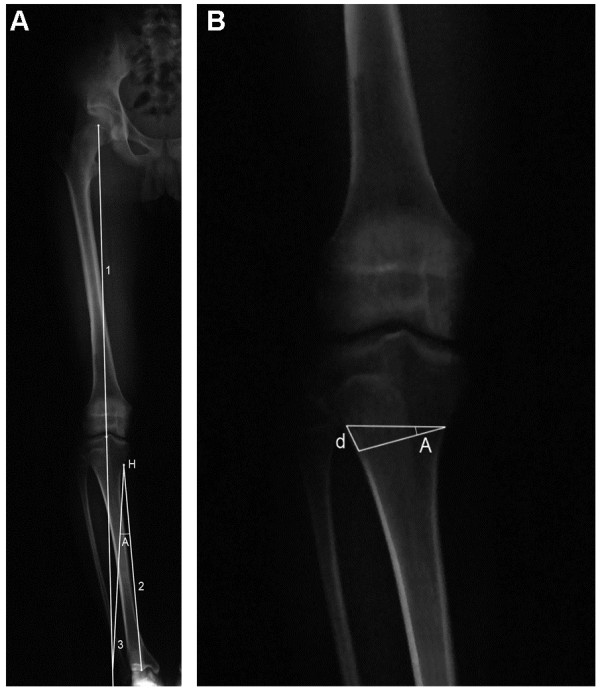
Miniaci method for frontal plane correction. (A)*Line 1* represents the planned Mikulicz line for the postoperative correction extending from the center of the hip through the center of the knee, past the ankle. *Line 2* connects the osteotomy hinge point (*H*) with the center of the ankle. The *H* point was located on the medial cortex of the proximal tibia at the lower level of tibial tuberosity. With *H* point as the center and the length of *line 2* as the radius, an angular arc is drawn from the center of the ankle to the intersection of *line 1. Line 3* connects the *H* point with the intersection of *line 1*. The angle formed by *lines 2* and *3* is the planned correction angle (*A*). (B)*Angle A* is now drawn on the prospective site of the osteotomy on the AP radiographs of the knee joint. The distance (*d*) of the two lines at the lateral cortex was measured as the osteotomy wedge base (magnification factor must be considered).
